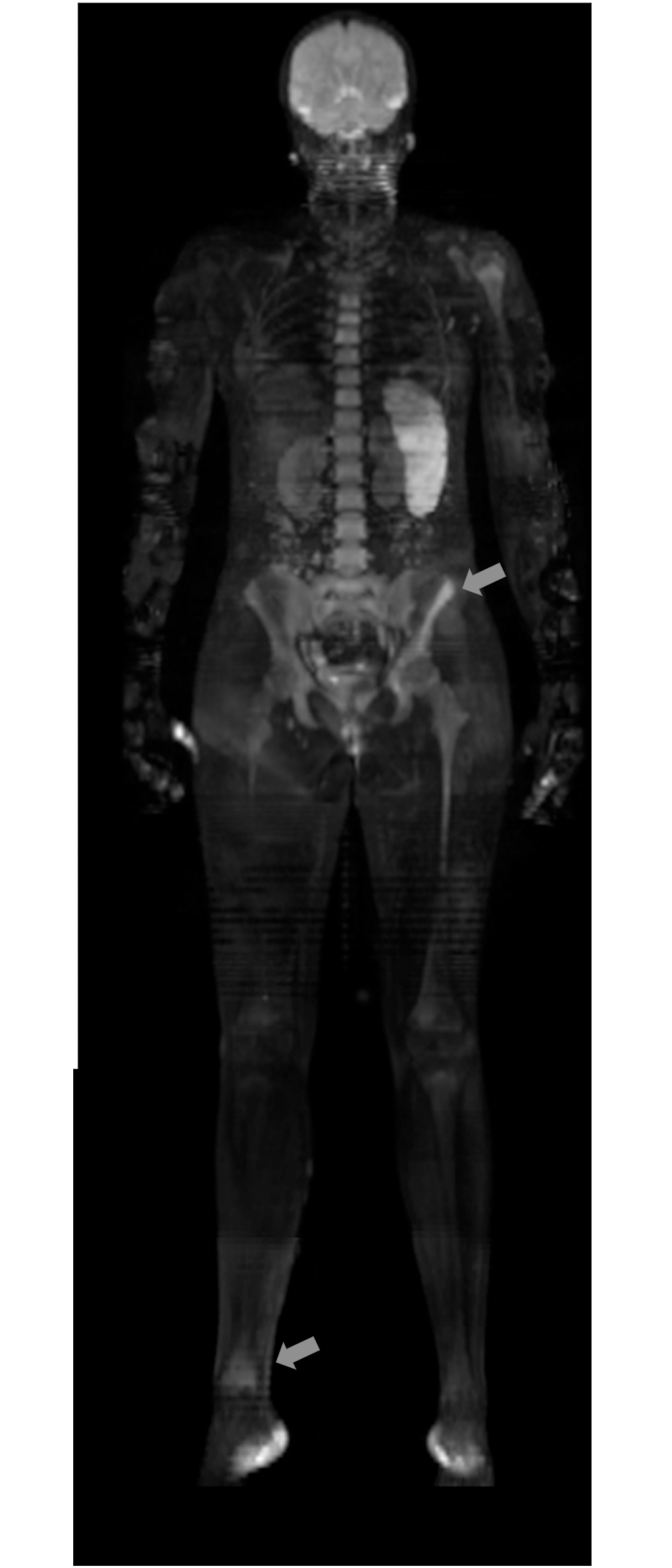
“At-a-glance” visualisation of Whole-body-DWI. Intuitive visualisation of Whole-body-DWI with maximum intensity projection MIP in a 12-year-old girl with multifocal CRMO manifestations shows signal alterations on DWI in the left pelvis (Os ilium) and in the right ankle/ distal tibia.
